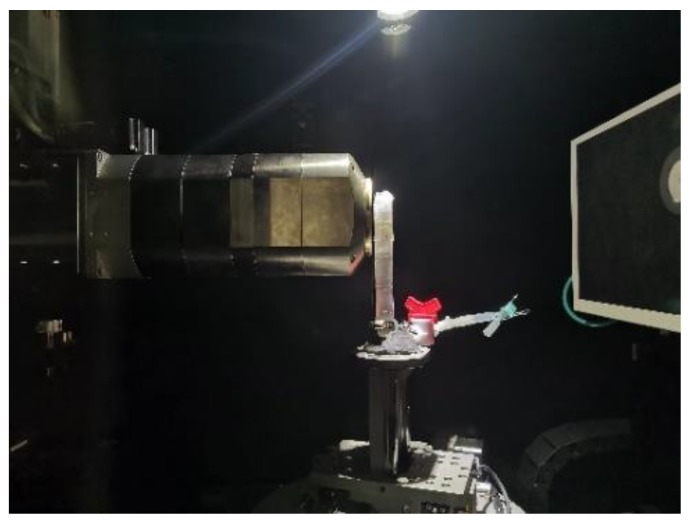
CT scan of the tailings sand sample.
